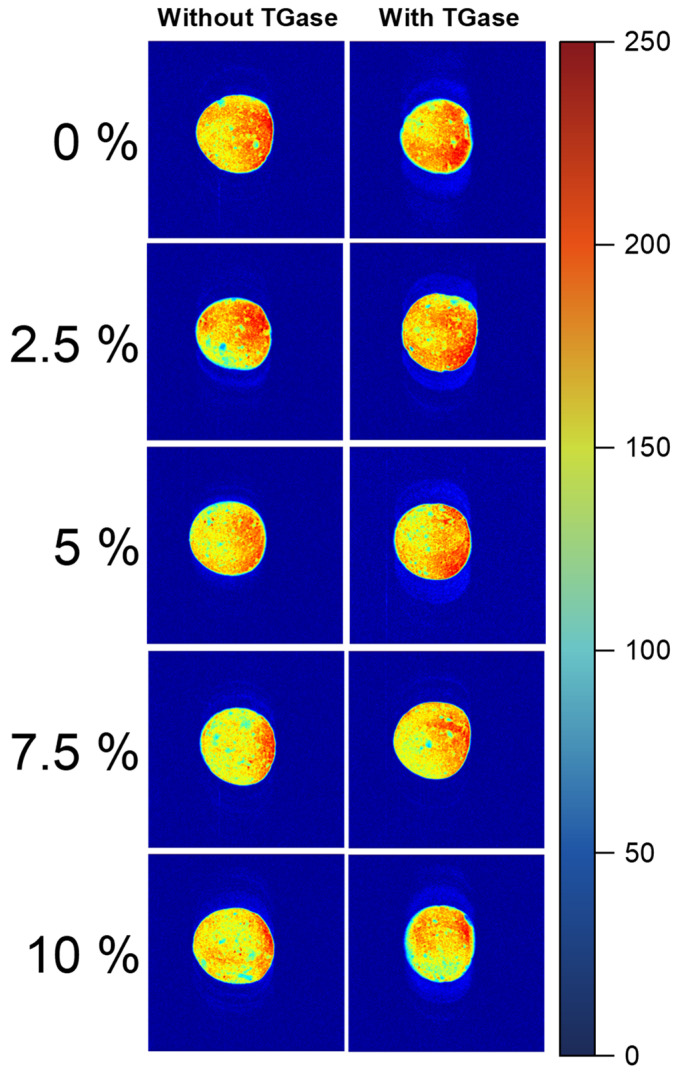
T2-weighted magnetic resonance images of surimi gel with different amounts of crayfish muscle (0%, 2.5%, 5%, 7.5% and 10%) in the samples with TGase and without TGase.
